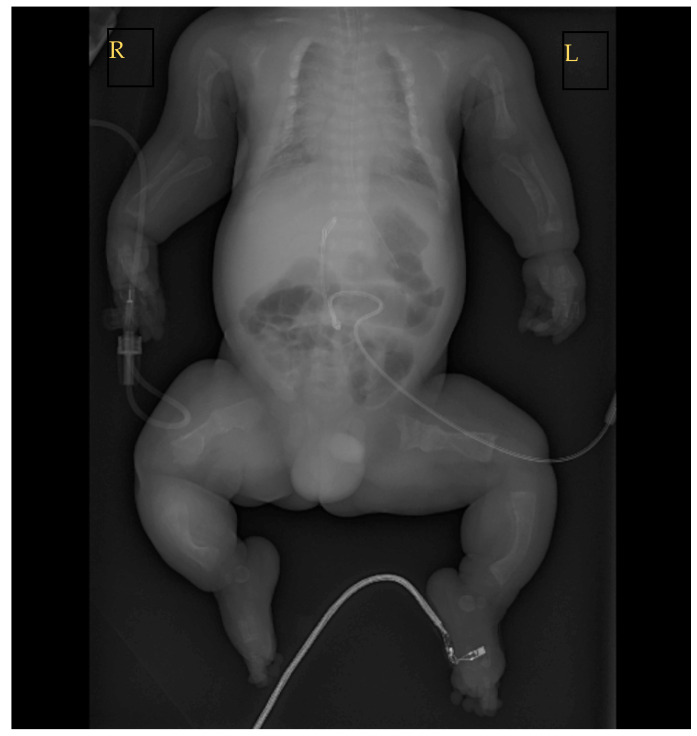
Chest, abdomen, and extremities X-ray of the lying newborn. Conclusions of the X-ray included: signs of skeletal dysplasia—multiple bone deformities with possible previous bone fractures in utero; fracture of the right ulna in the middle third; and a deformed and narrow chest cavity with incompletely expanded lungs. R—right side, L—left side.
